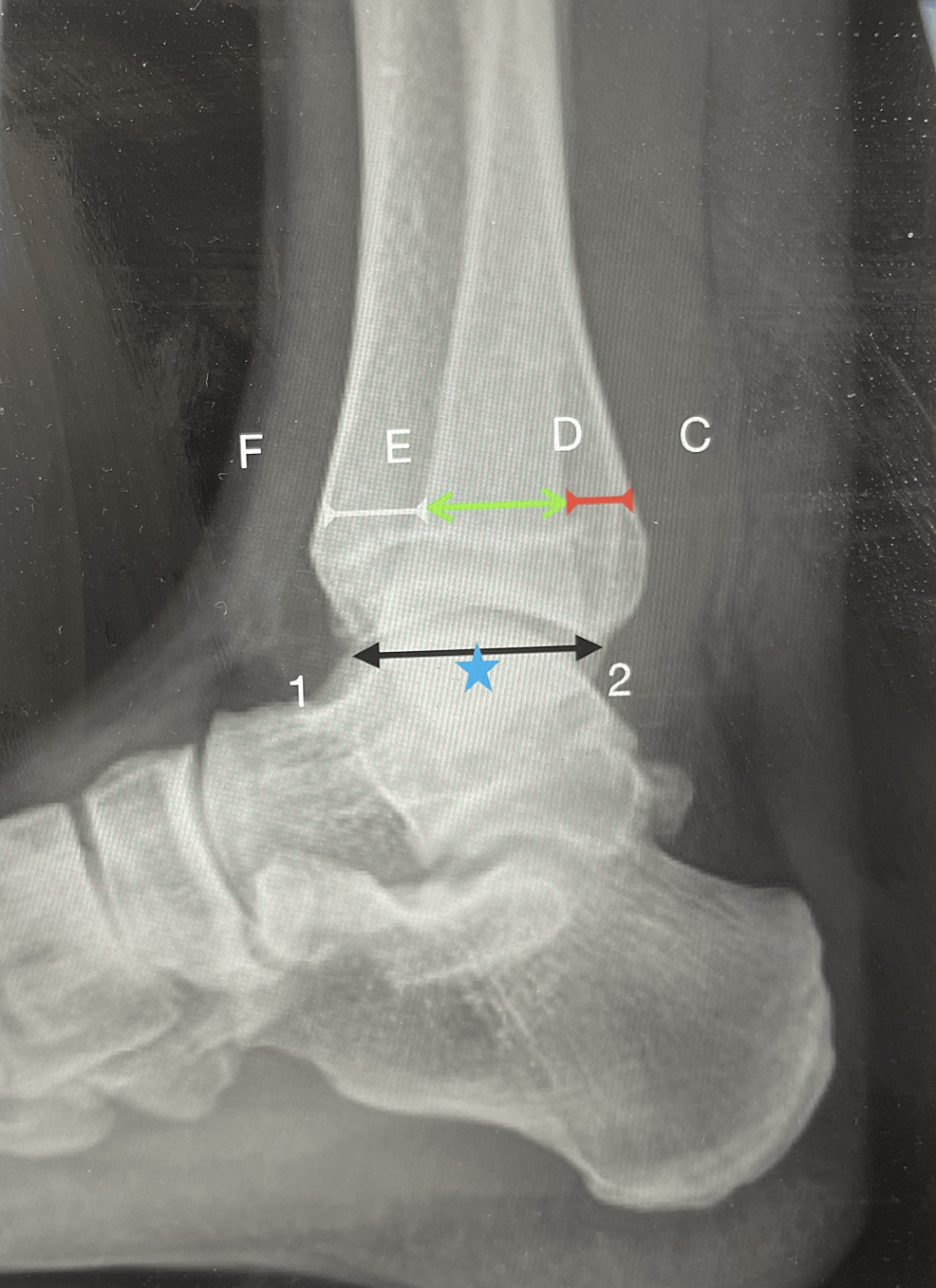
Example of radiographic analysis F-E indicates the anterior tibiofibular interval, E-D indicates the fibular width, D-C shows the posterior tibiofibular interval, 1-2 indicates the tibial plafond, * indicates the midpoint; all measurements were performed 1 cm above the midpoint.
